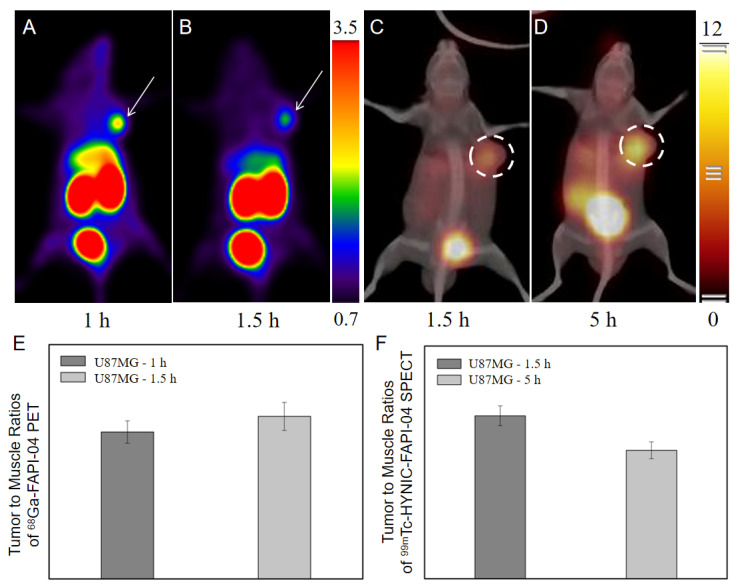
^68^Ga-FAPI-04 PET images of FAP-positive tumors at different time point (A,B) and ^99m^Tc-HYNIC-FAPI-04 SPECT images of FAP-positive tumors at different time point (C,D). The comparison of ratios of tumor to muscle between 1.5 h P.I. and 5 h P.I. in ^68^Ga-FAPI-04 PET (E) and ^99m^Tc-HYNIC-FAPI-04 SPECT (F). The white arrow and white dotted circles all pointed to FAP-positive tumors.
